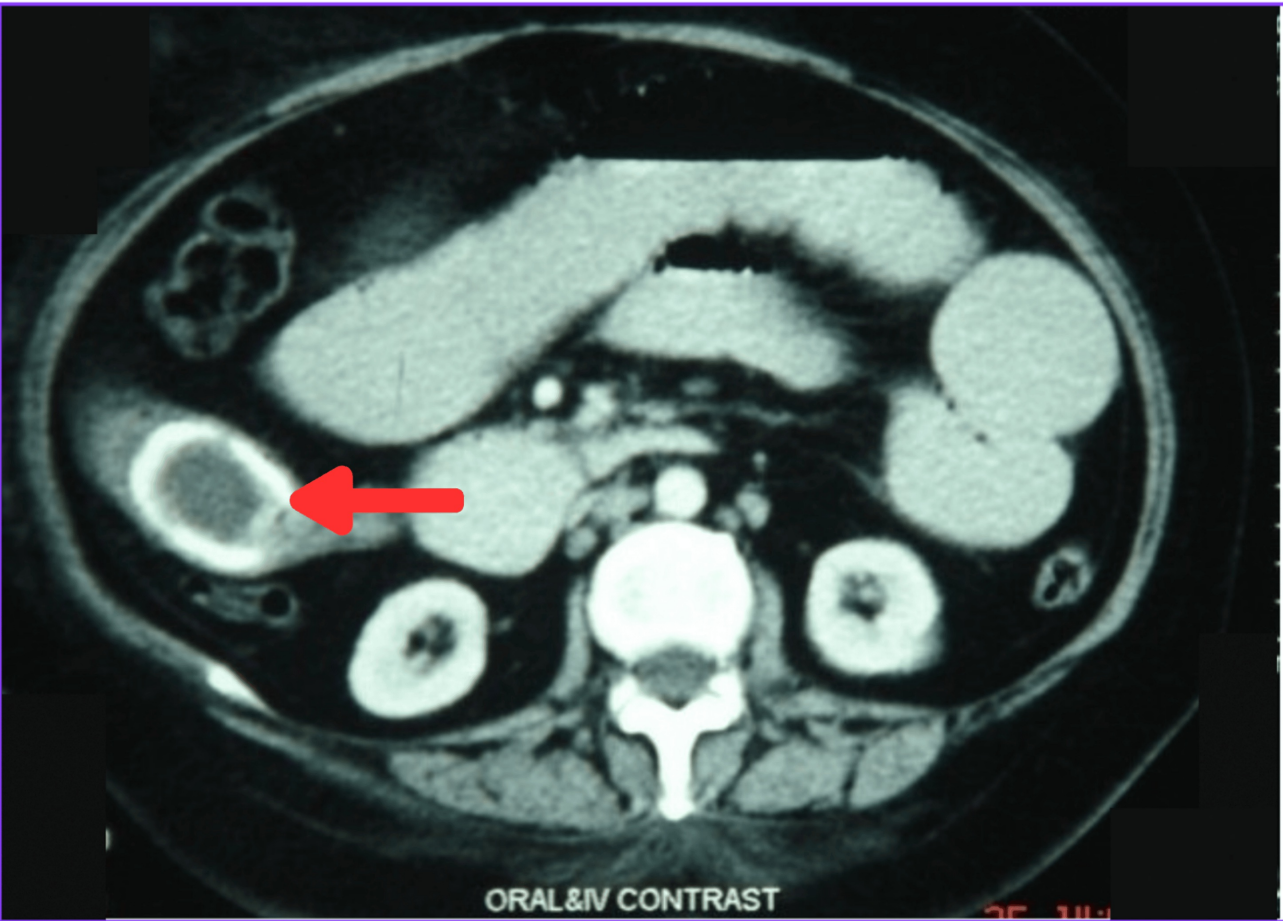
CT scan of the abdomen with contrast shows an impacted stone in the distal ileum.
